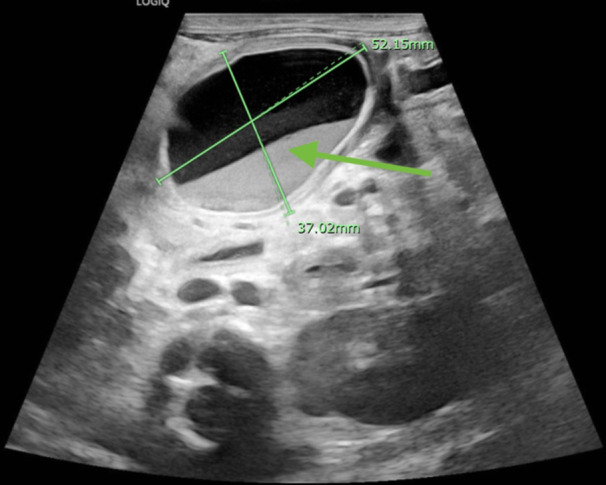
Ultrasound #2 5.2 × 3.7 cm cystic structure with fluid debris (arrow) and double mucosa sign.
